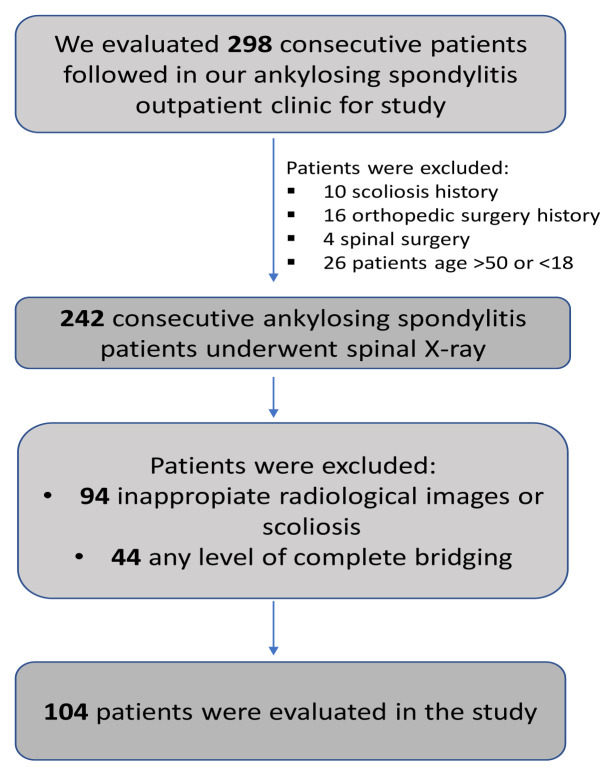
Lateral whole-spine radiograph of a patient with ankylosing spondylitis.
